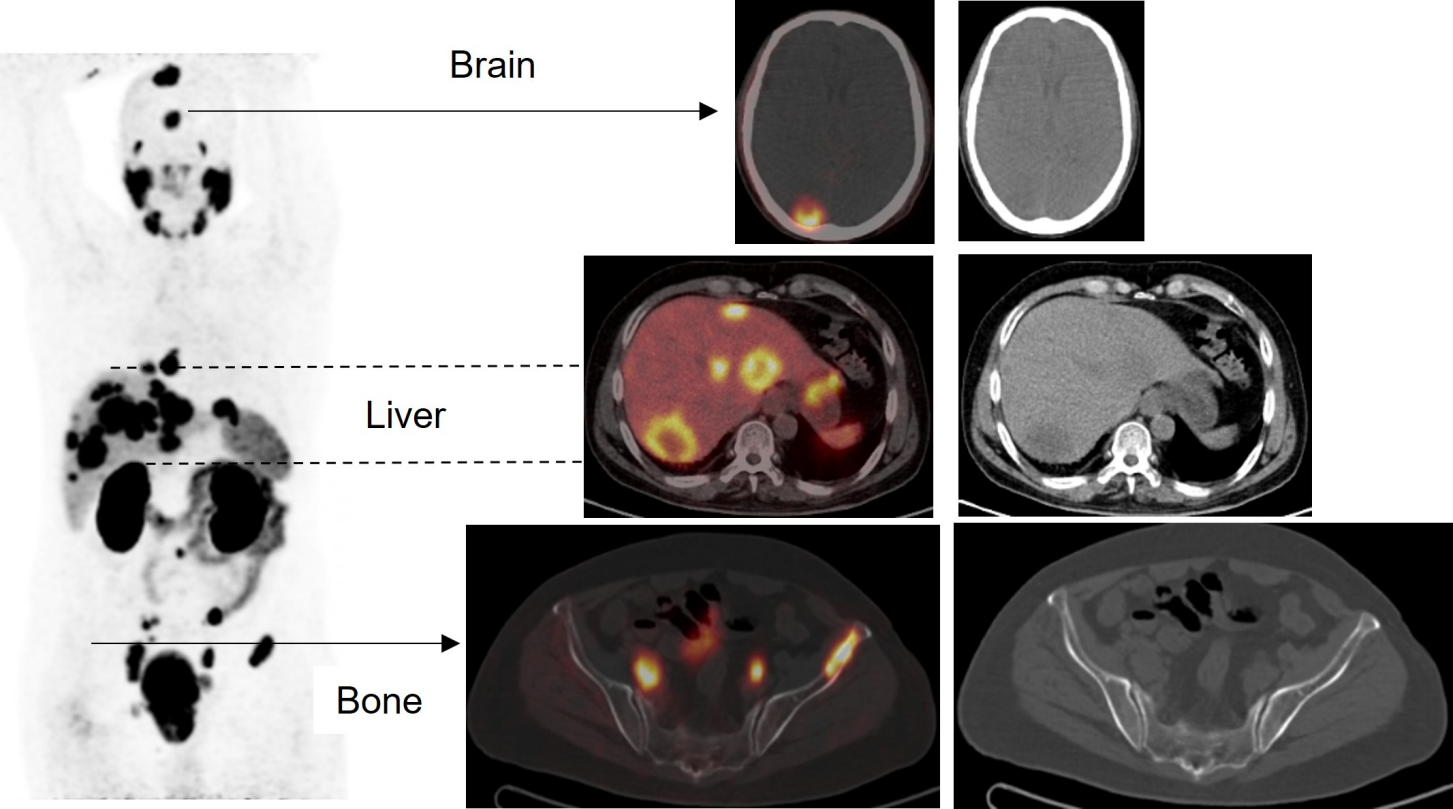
A new case of prostate cancer (poorly differentiated carcinoma) with a PSA level of 21 has been referred for staging. The staging evaluation revealed the presence of multiple lymph nodes with PSMA uptake in the para-aortic, common iliac, external, and internal iliac chains, measuring up to 28mm with a SUV_max_ of 15.10. Additionally, the liver exhibited multiple PSMA-avid masses in both lobes, measuring up to 52mm with an SUV_max_ of 16.16. The prostate gland appeared enlarged and lobulated, displaying significant inhomogeneous PSMA uptake. There is a likelihood of invasion into the infero-posterior wall of the urinary bladder, with an SUV_max_ of 18.07. Metastases were observed in various locations, including the skeletal system, both liver lobes, bilateral lung field, and brain. Notably, there was intense uptake in the salivary glands.
